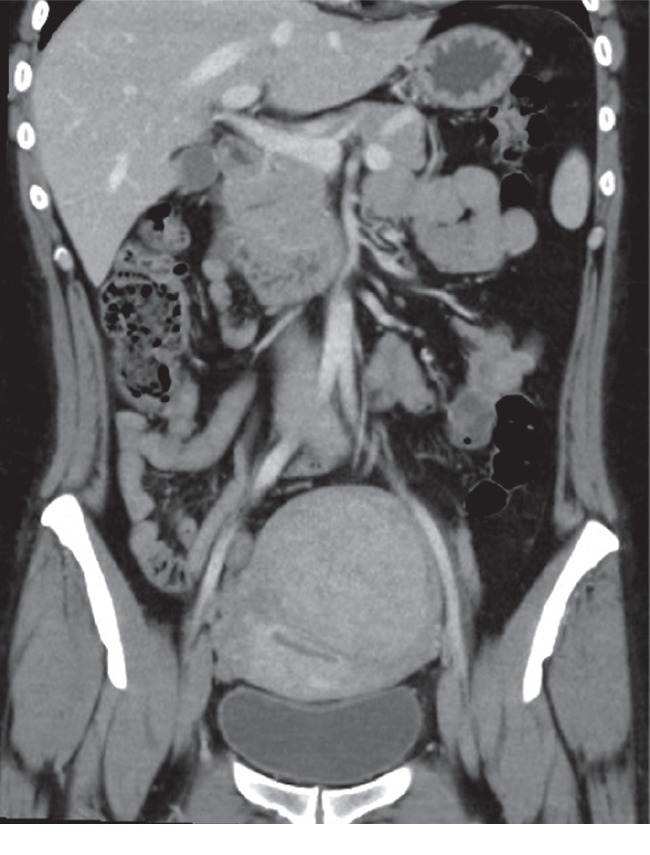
CT scan of abdomen (coronal plane) showing the intramural uterine fibroid.
